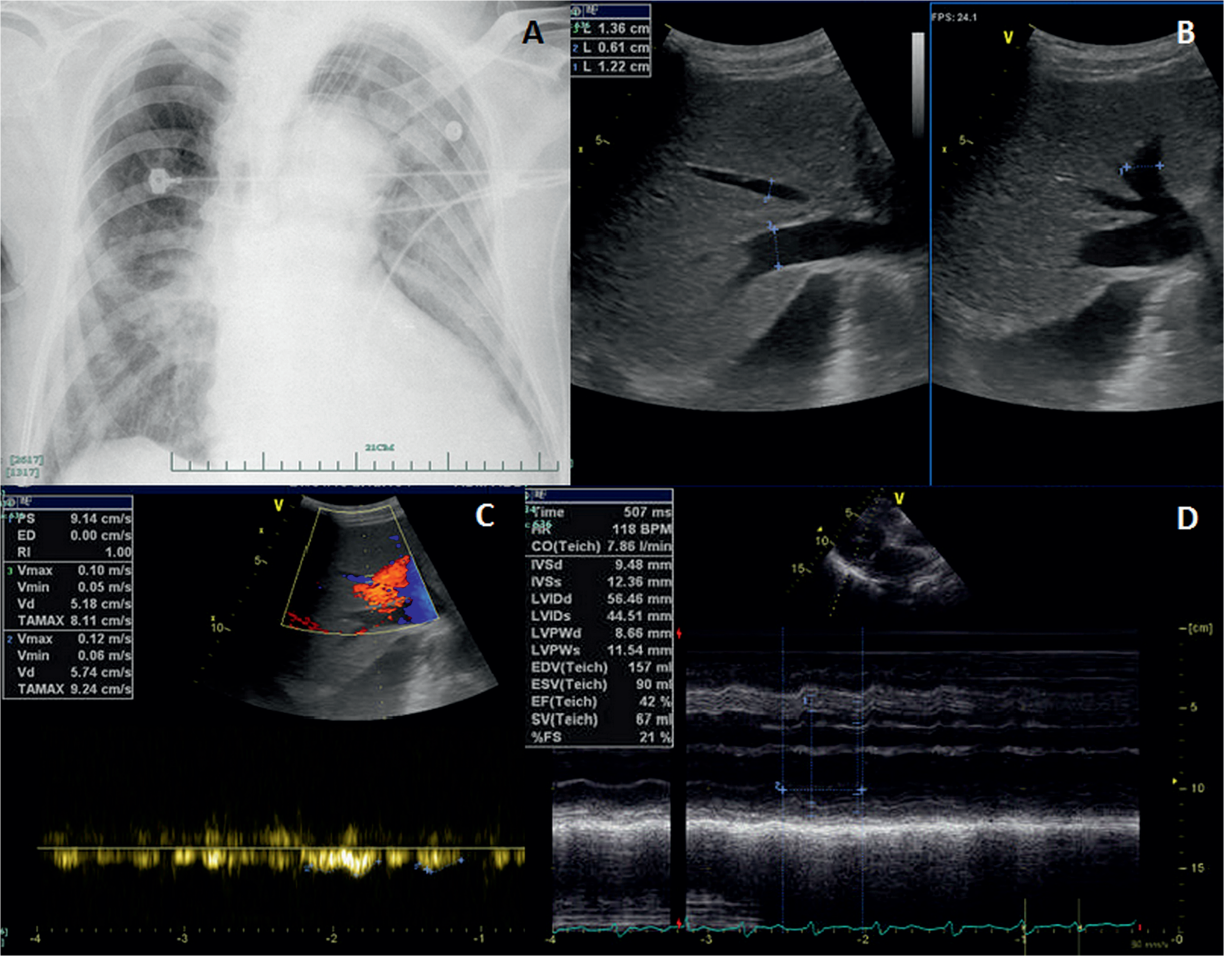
Radiographic and ultrasound findings in case 1. (A) Chest radiograph at the time of admission showed bilateral pulmonary infiltration with consolidation. (B) Ultrasound image showed a dilated hepatic vein (left branch, 1.22 cm; middle branch, 0.61 cm and right branch, 1.36 cm). (C) Ultrasound image showed slowed hepatic vein flow (middle branch, 9.14 mm/s). (D) Echocardiogram showed a dilated left ventricle (LVIDd 56.46 mm) and cardiac failure (LVEF 42%) with weakened left-ventricular wall motion. LVEF, left ventricular ejection fraction; LVIDd, left ventricular internal diameter at end-diastole.
